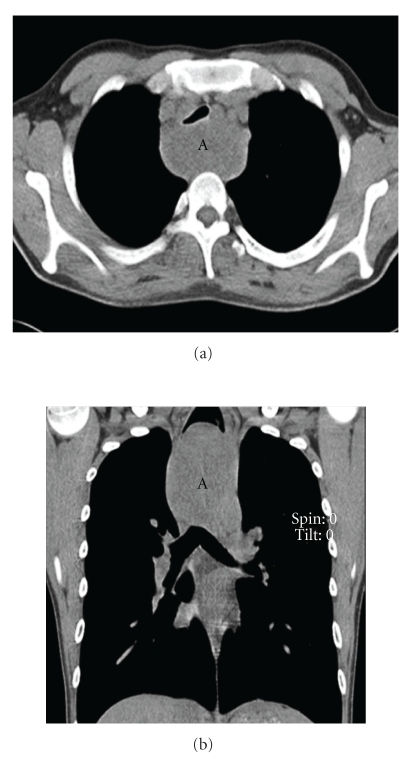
CT scan (a) with coronal view (b) showing large posterior mediastinal mass (A) with tracheal compression.
